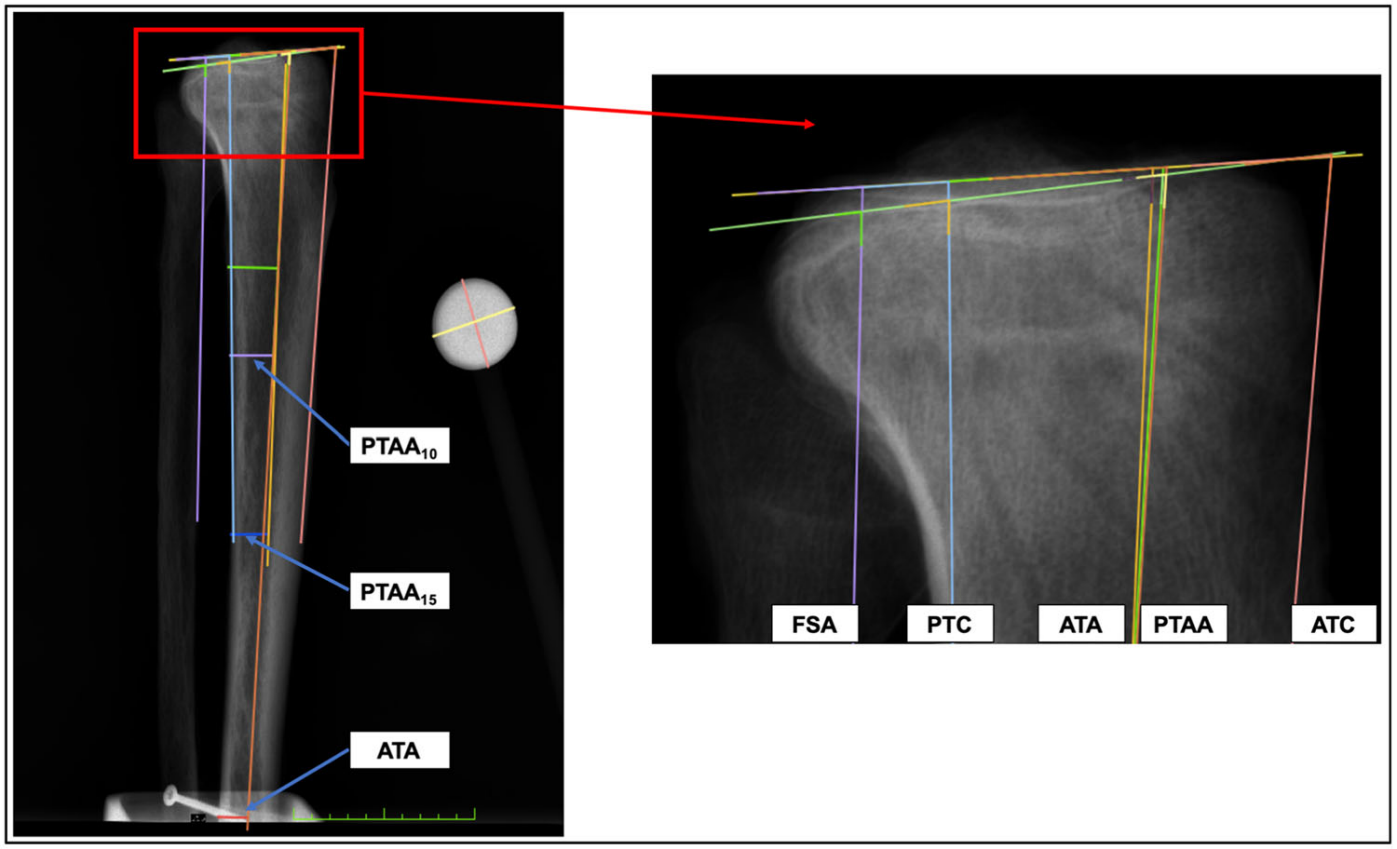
True lateral radiograph of a left tibia. The posterior tibial slope was defined as the angle between the line perpendicular to six different anatomical references and the line tangent to the medial and lateral tibial plateau. ATA, anatomical tibial axis (orange), line connecting the midpoints of the outer cortical diameter at 5 cm distal and maximum length of the tibia; ATC, anterior tibial cortex (red); PTAA_10_, proximal tibial anatomical axis (yellow), line connecting the midpoints of the outer cortical diameter at 5 and 10 cm distal to the tibial tuberosity; PTAA_15_, proximal tibial anatomical axis (green), line connecting the midpoints of the outer cortical diameter at 5 and 15 cm distal to the tibial tuberosity; PTC, posterior tibial cortex (blue); FSA, fibular shaft axis (violet).
